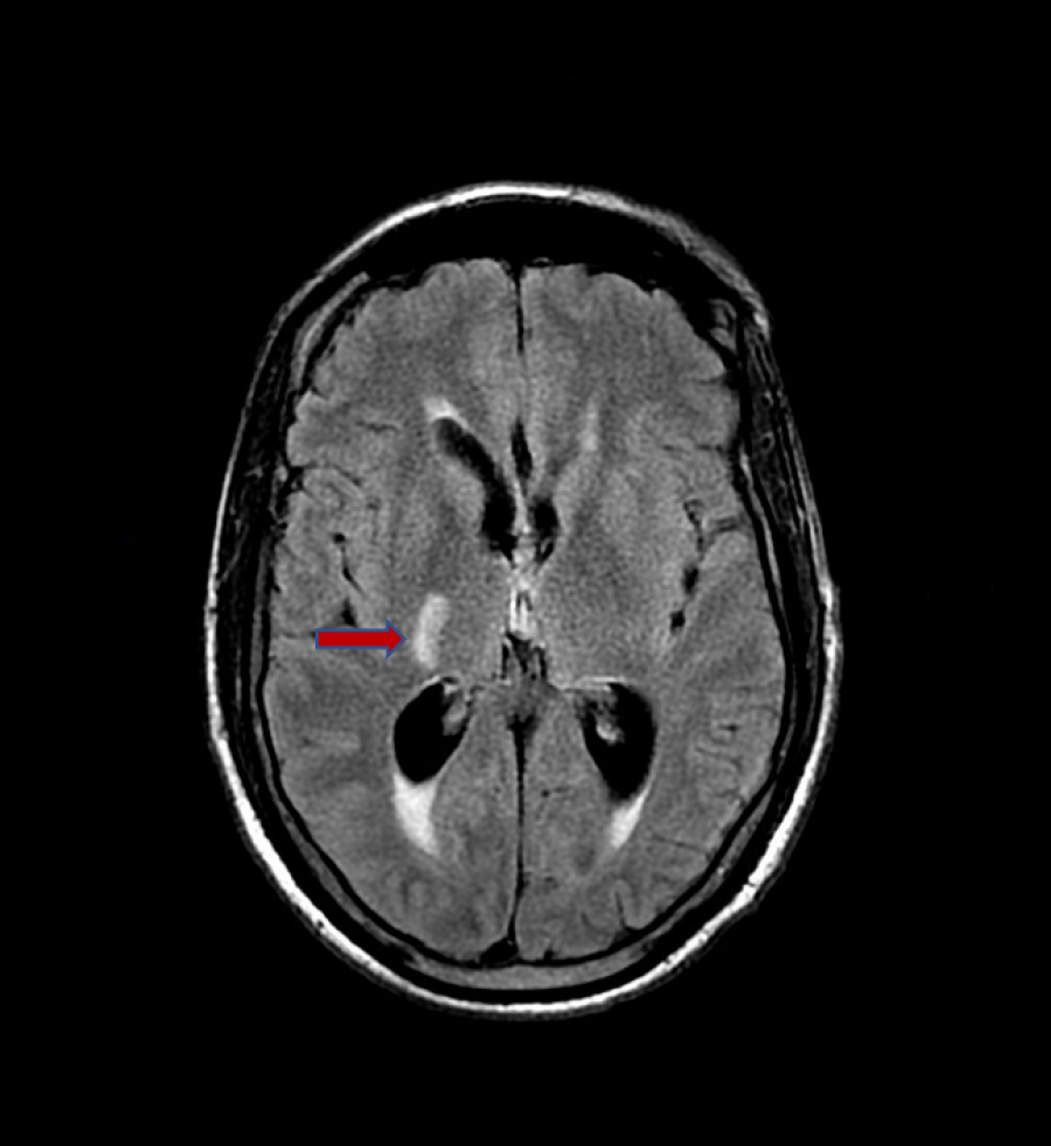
Magnetic resonance images of the brain with fluid-attenuated inversion recovery showing right basal ganglia lesion Red arrow indicates cryptococcomas lesion.
